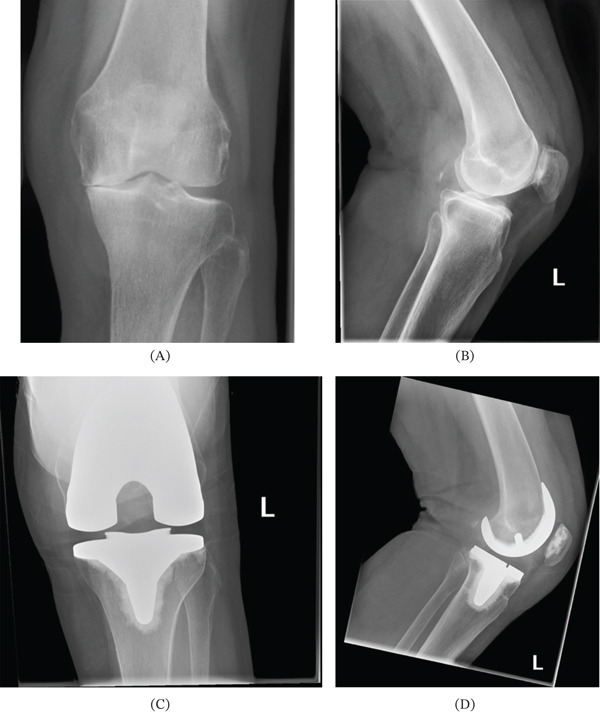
Plain radiographs of the left knee. Anteroposterior (A) and lateral (B) views pre‐TKR show Grade 4 medial compartment osteoarthritis and Grade 3 changes in the lateral compartment. Rosenburg (C) and lateral (D) views post‐TKR show a fully cemented fixed bearing primary TKR in‐situ.
